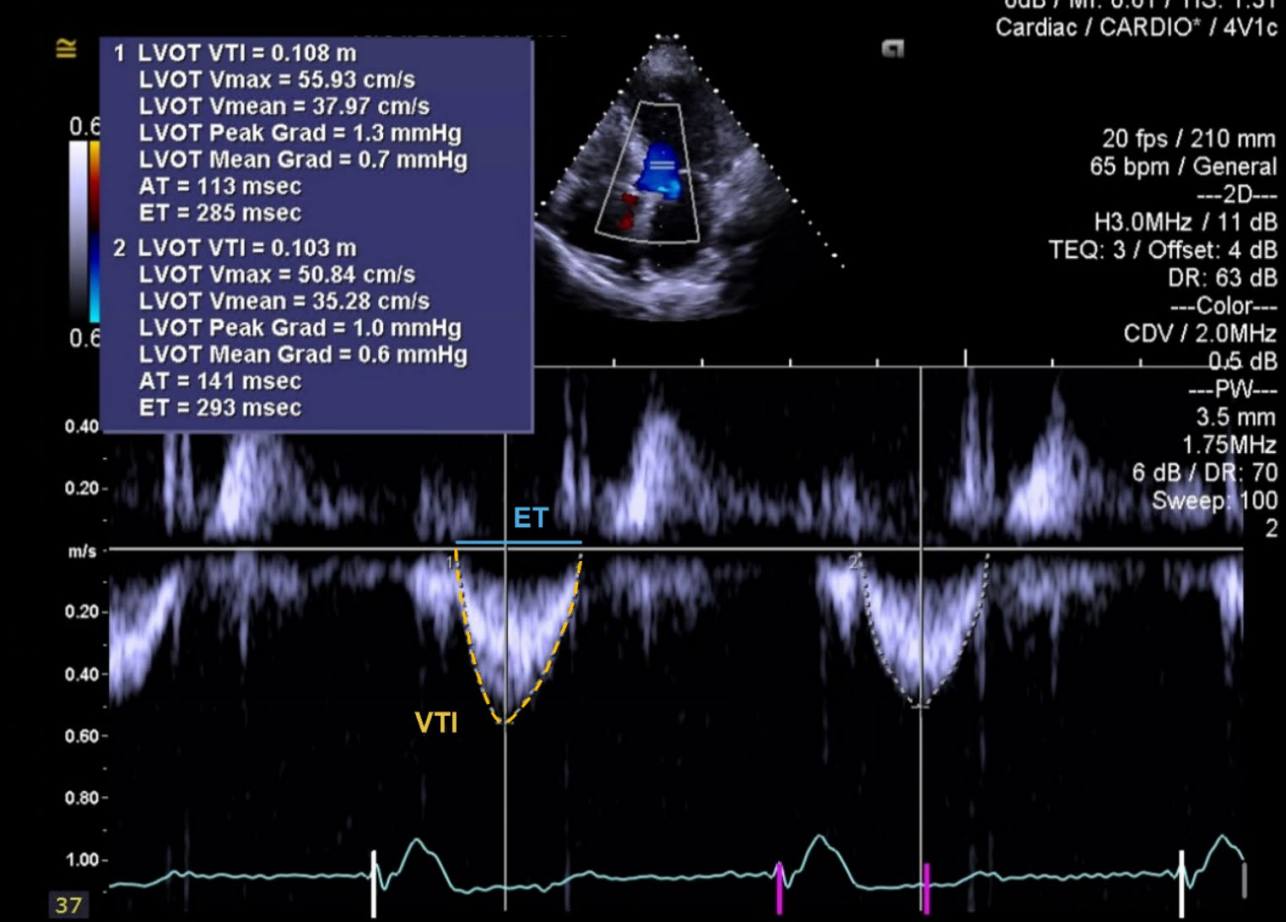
Pulse-wave Doppler in the left ventricle outflow tract (LVOT) of a patient with severe aortic stenosis. Transaortic flow rate (FR) is calculated dividing the Doppler-derived stroke volume by the ejection time (ET, in blue), using velocity time integral (VTI, in yellow) of the LVOT and respective LVOT diameter (LVOTd, not shown)
